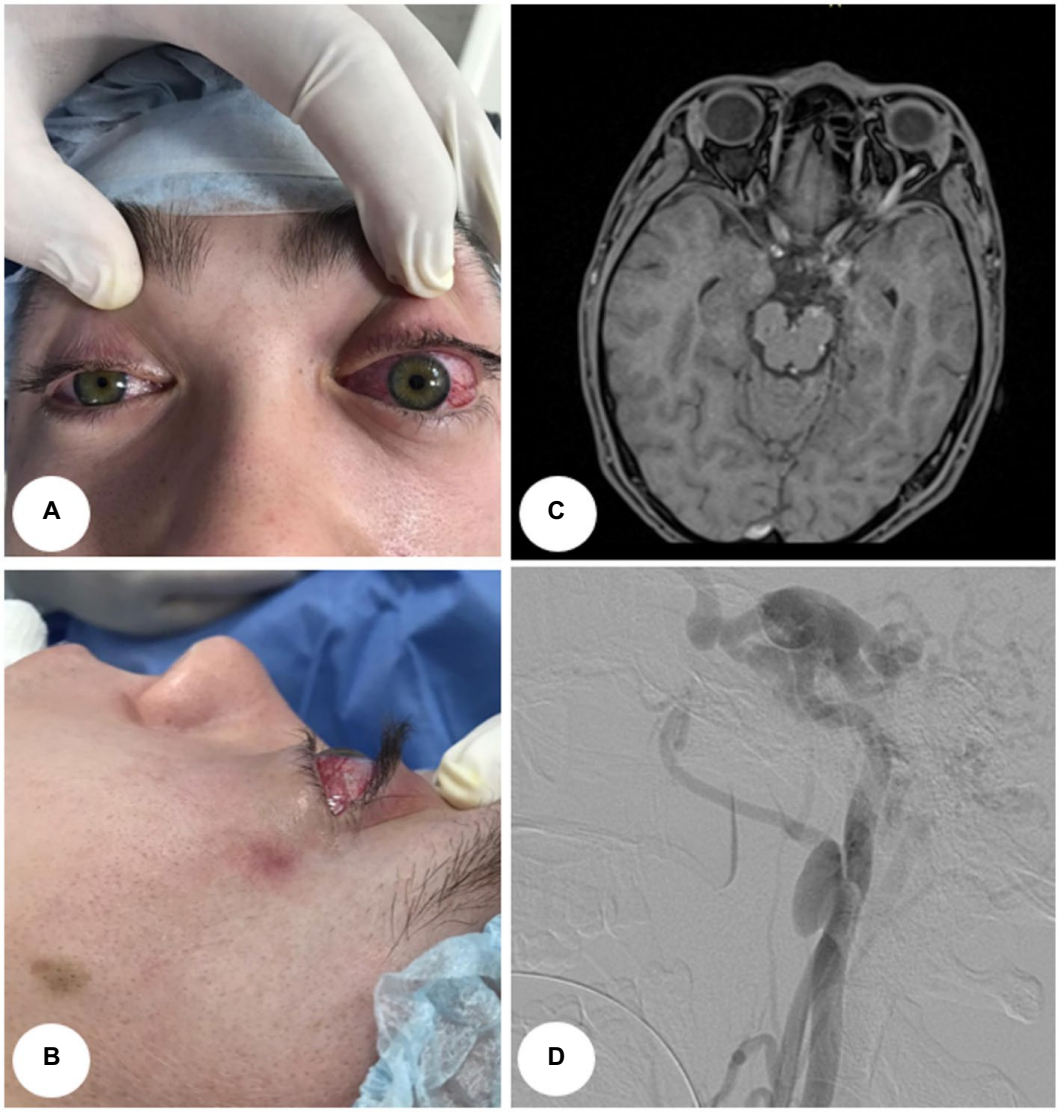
A visual manifestation and instrumental examinations of Case 1. (A) Anterior view. (B) Lateral view. (C) Axial MRI shows dilatation of the superior ophthalmic vein in a patient with left side carotid-cavernous fistula. (D) Digital subtraction angiogram (lateral view) shows a dural carotid-cavernous fistula drained anteriorly and posteriorly with a combination of extra cranial internal carotid aneurysm.
